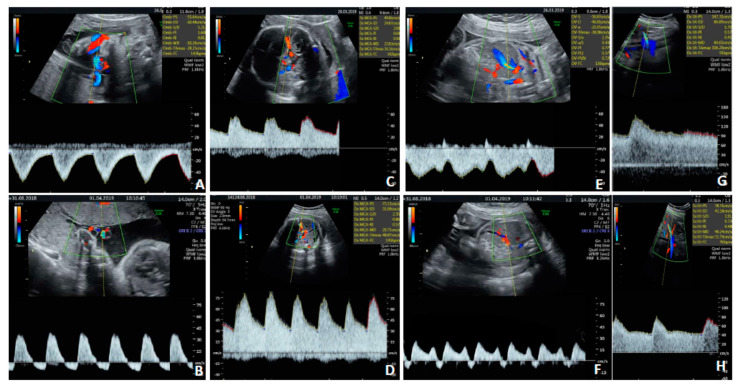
Prenatal Doppler findings for Case 2, showing the rapid progression of fetal Doppler alterations due to CMV infection. Umbilical artery Doppler at diagnosis of fetal growth restriction (A) and at delivery 4 days later (B). Middle cerebral artery Doppler at diagnosis (C) and at delivery (D). Ductus venosus Doppler at diagnosis (E) and at delivery (F). Uterine artery Dopplers were both normal (G,H).
